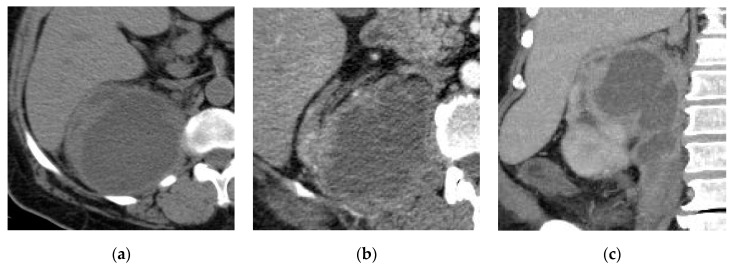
Right renal abscess. (a) Axial non-contrast CT image shows right upper polar cystic lesion with a thick wall. (b) Axial and (c) coronal reformatted post-contrast CT images show upper polar abscess extending to the right psoas muscle with a thick enhancing wall.
